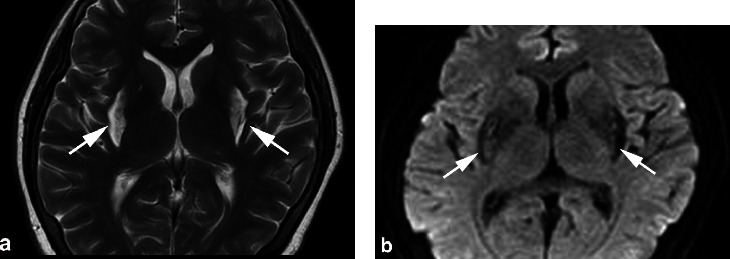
MRI identified putaminal lesions in patients with methanol-induced optic neuropathy. Brain MRI images of patient 7, 116 days after acute onset of methanol poisoning, (a) axial T2 image demonstrating hyperintense signals (arrows) in bilateral putamina, and (b) DWI b = 1000 image demonstrating dominant low signal and partial high signal in the putaminal lesion.
